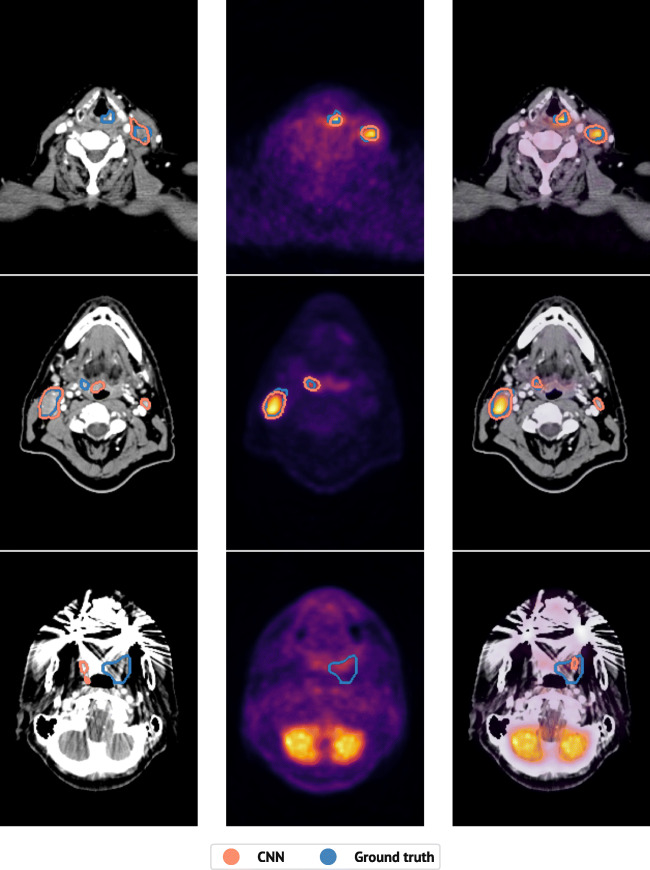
The predicted (*red*) and true (*blue*) delineations for one representative image slice from three different patients in the test cohort. From left to right: CT-based predictions; PET-based predictions; PET/CT-based predictions. From top to bottom, different subjects for whom an experienced oncologist gave the PET/CT-based predictions a qualitative score of 10, 8 and 2, respectively. Performance metrics for the shown patients are marked with italicised text in Table 3. Animations are given in Online Resources 2 (top patient), 3 (middle patient) and 4 (bottom patient)
